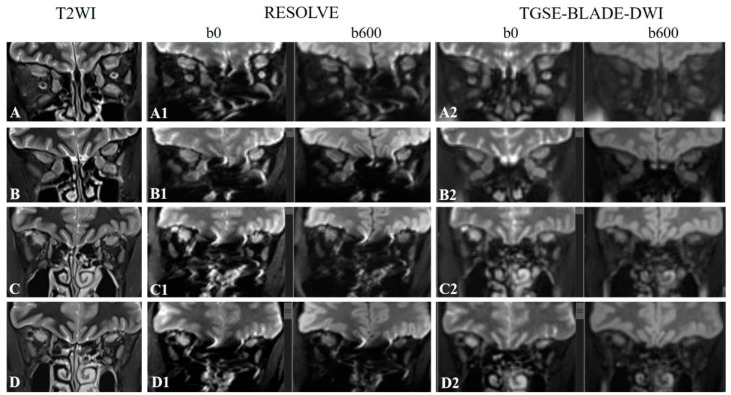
Representative coronal T2-weighted imaging (T2WI) images (A–D), coronal RESOLVE DWI b0 and b600 images (A1–D1), and coronal TGSE-BLADE-DWI b0 and b600 images (A2–D2) of a 54-year-old female patient with CAS = 4 (A, A1, A2, and B, B1, B2) and a 50-year-old male patient with CAS = 3 (C, C1, C2 and D, D1, D2). For slice 1 (A, A1 and A2), the subjective scores for the right superior rectus, right inferior rectus, right medial rectus, right lateral rectus, left superior rectus, left inferior rectus, left medial rectus, and left lateral rectus were 3, 2, 2, 2, 3, 2, 1, and 2 for RESOLVE and 3, 2, 3, 1, 3, 3, 2, and 1 for TGSE-BLADE-DWI, respectively. For slice 2 (B, B1 and B2), the subjective scores for the EOMs were 3, 3, 2, 1, 3, 1, 1, and 1 for RESOLVE and 3, 3, 3, 1, 3, 3, 3, and 2 for TGSE-BLADE-DWI, respectively. For slice 3 (C, C1 and C2), the subjective scores for the EOMs were 3, 1, 1, 3, 3, 1, 2, and 3 for RESOLVE and 3, 2, 2, 1, 3, 3, 3, and 3 for TGSE-BLADE-DWI, respectively. For slice 4 (D, D1 and D2), the subjective scores for the EOMs were 3, 1, 1, 2, 3, 1, 1, and 3 for RESOLVE and 3, 1, 1, 1, 3, 3, 3, and 2 for TGSE-BLADE-DWI, respectively.
